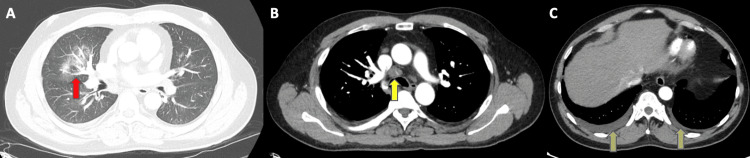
Computed tomography angiography. Computed tomography angiography of the chest demonstrating patchy areas of consolidation (red arrow), extensive mediastinal (pretracheal) lymphadenopathy (yellow arrow), and bilateral pleural effusions (green arrows).
